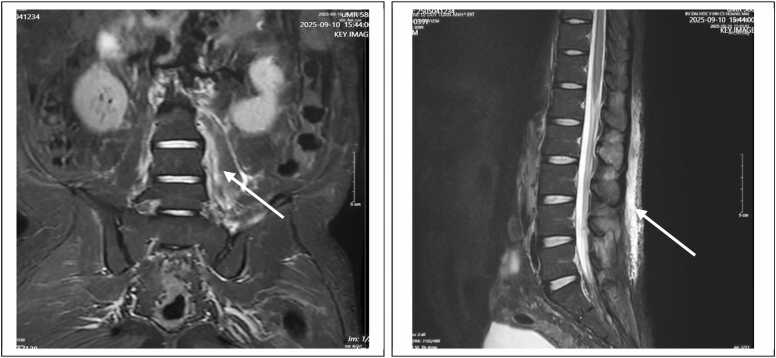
Contrast- enhanced MRI of the lumbar spine showing a diffuse edema with increased muscle volume and signal intensity was observed in the bilateral paraspinal muscles, iliopsoas muscles, and the lumbar paraspinal soft tissues (white arrow).
